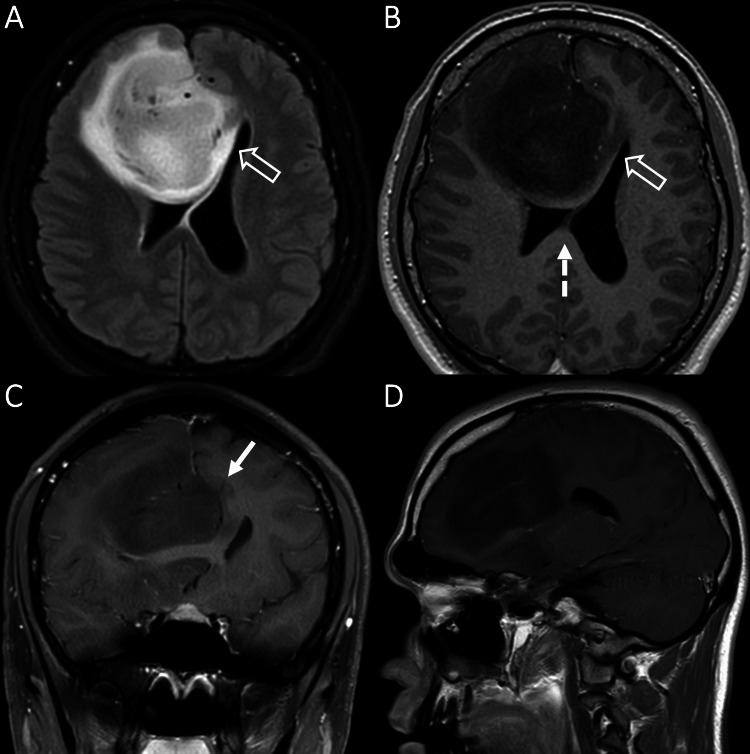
The patient’s baseline MRI showing a large right frontal oligodendroglioma, WHO grade III A, an axial MRI image showing a 6.2 cm solid, FLAIR, hyperintense mass centered at the right frontal pole. T1 post-contrast MRI images in B-D (B axial, C coronal, D sagittal) demonstrate T1 hypointensity with no significant enhancement. The mass causes subfalcine herniation (solid arrow in C) and there is an extension of white matter signal abnormality across the corpus callosum, across the midline to the left (open arrows in A, B). FLAIR: fluid-attenuated inversion recovery
